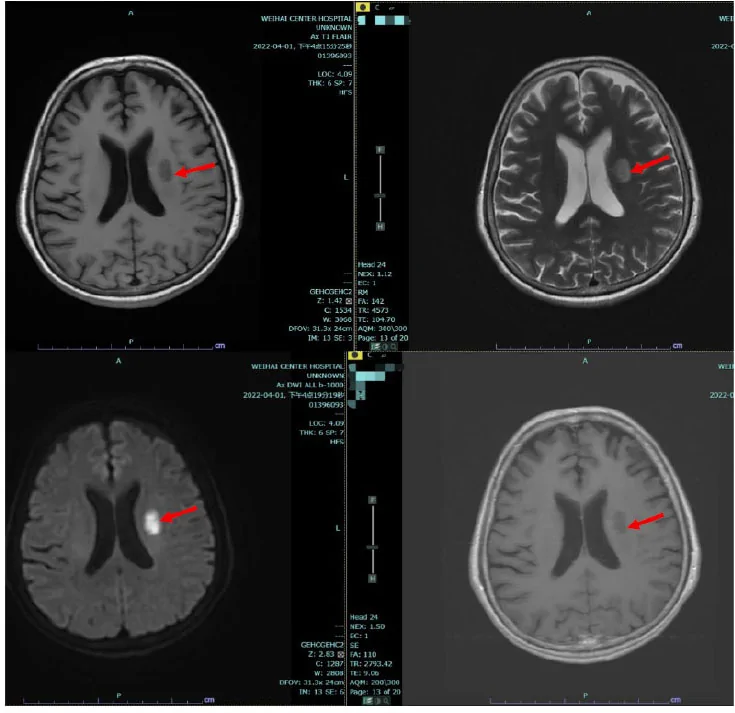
Brain MRI from April 1, 2022, shows patchy areas of prolonged T1 and T2 signal intensities in the left basal ganglia, with high signal on DWI and no significant enhancement on T1 post-contrast.
